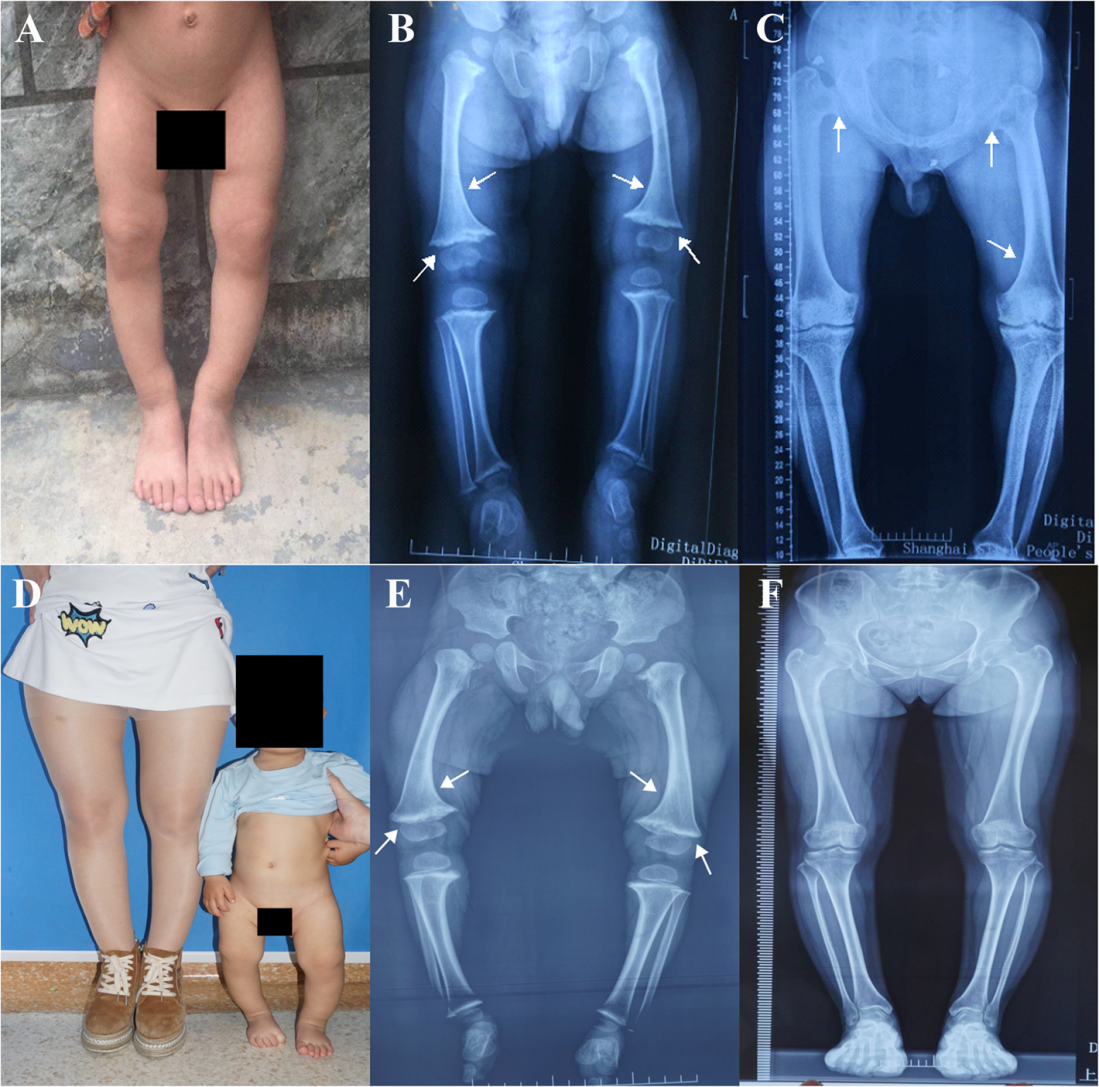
The clinical and radiological features of patients in two affected families. The legs of proband in family 1 exhibited evident “O” shape (a) and bowing of the femurs and widening and irregularity of the growth plates of distal femur (white arrows) are shown in X-ray image (b). Moreover, the X-ray image of III:1 in family 1 also presented bowing of the femurs, deformity of growth plates and hips (white arrows) (c). In family 2, the proband showed deformity of the lower extremities (d) and similar radiological presentation (white arrows) (e) to proband in family 1. However, the proband’s mother in family 2 exhibited normal appearance in X-ray image (f)
